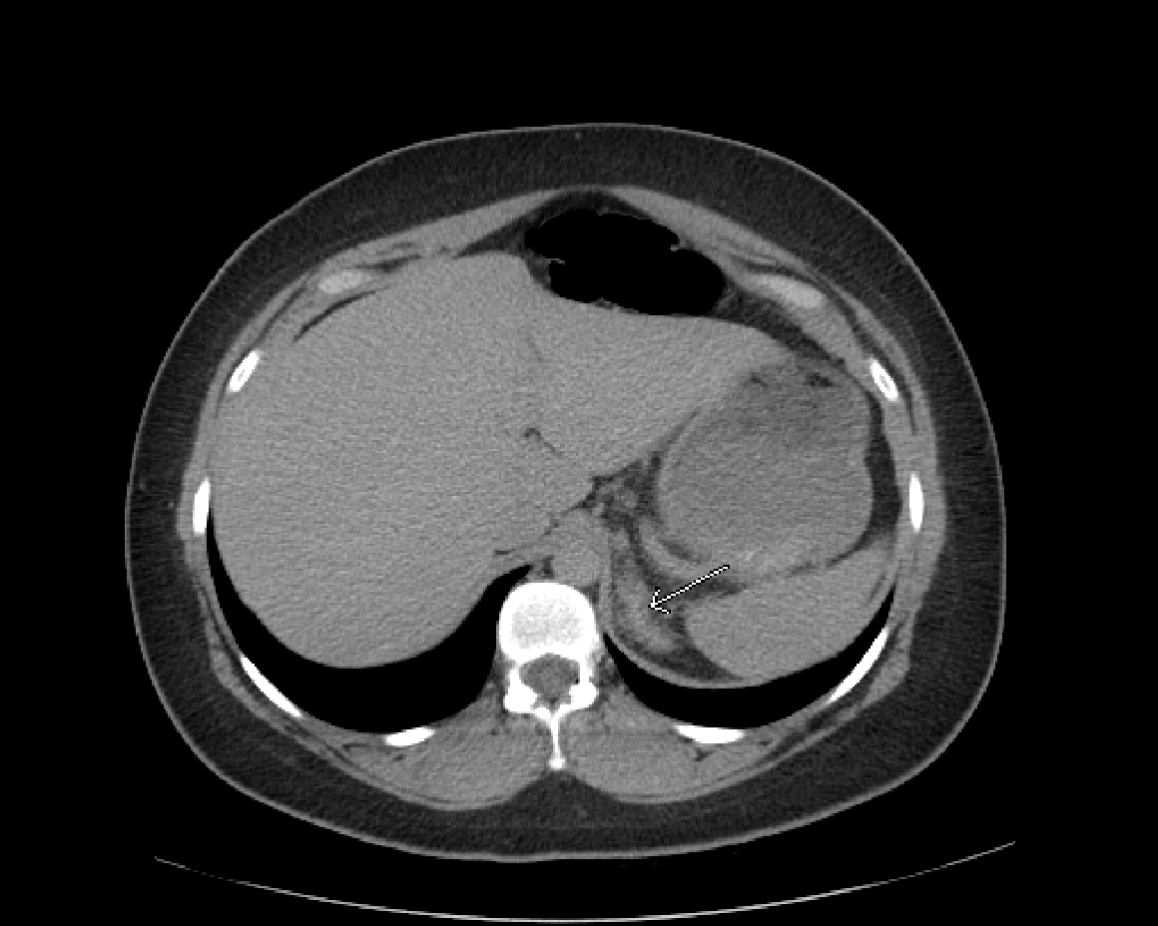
Cross-sectional abdominal CT scan with hyperattenuation of the left adrenal gland consistent with adrenal hemorrhage (arrow)
